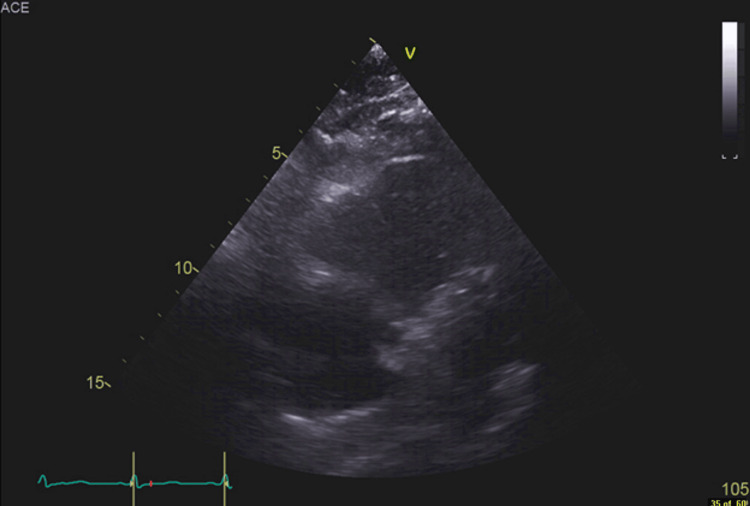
Apical view of the transthoracic echocardiogram showing a dilated right ventricle suggestive of right ventricular strain.
